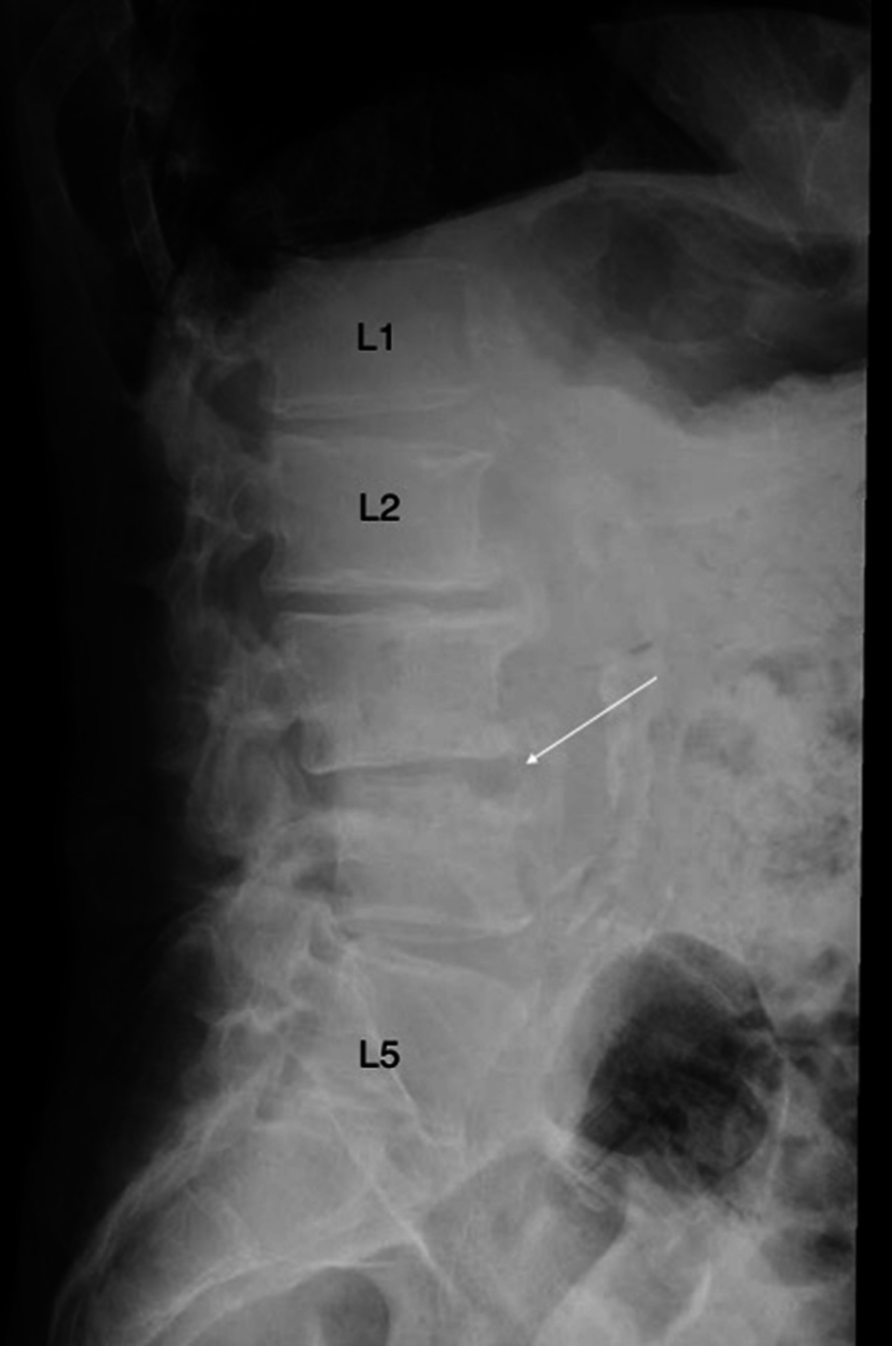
Lateral X-ray of the lumbar spine. There is evidence of erosive change on the anterior and superior plate of L4 that was not previously present. There is loss of disc height at L3-L4 and grade 1 anterolisthesis of L4-L3, findings suggestive of osteomyelitis and discitis.
